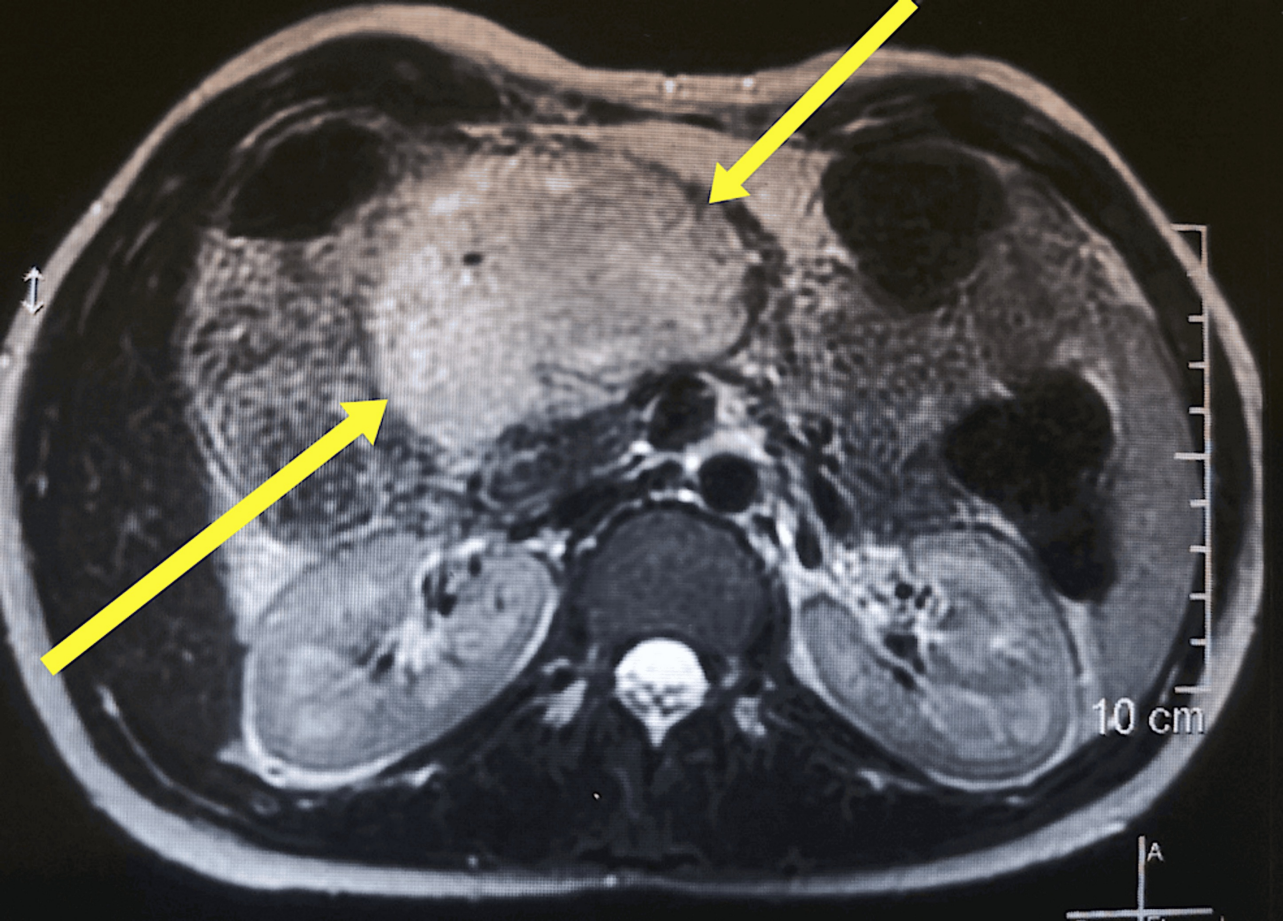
MRI aspect of the large solid-cystic mass of the head and pancreatic isthmus (yellow arrows)
